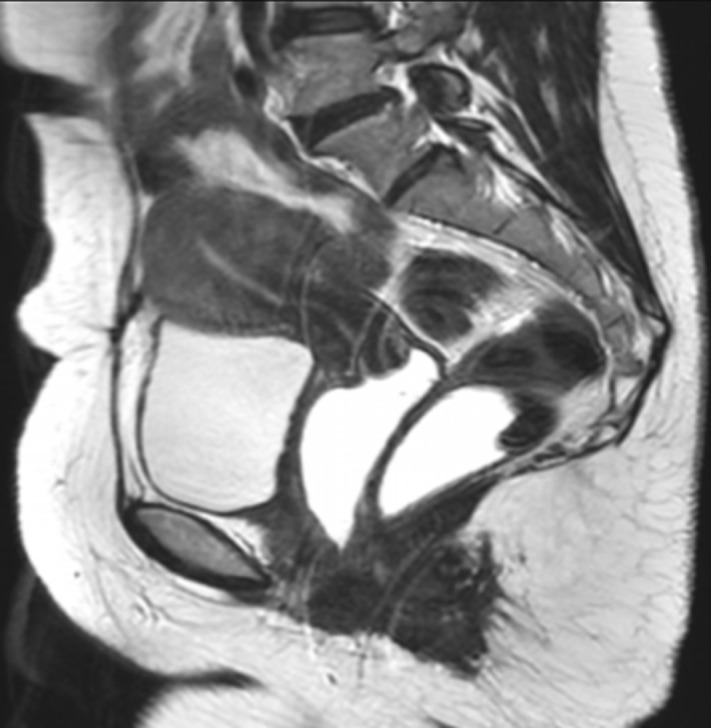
the sagittal T2-weighted sequence revealed no evidence of deep infiltrating endometriosis or adenomyosis
